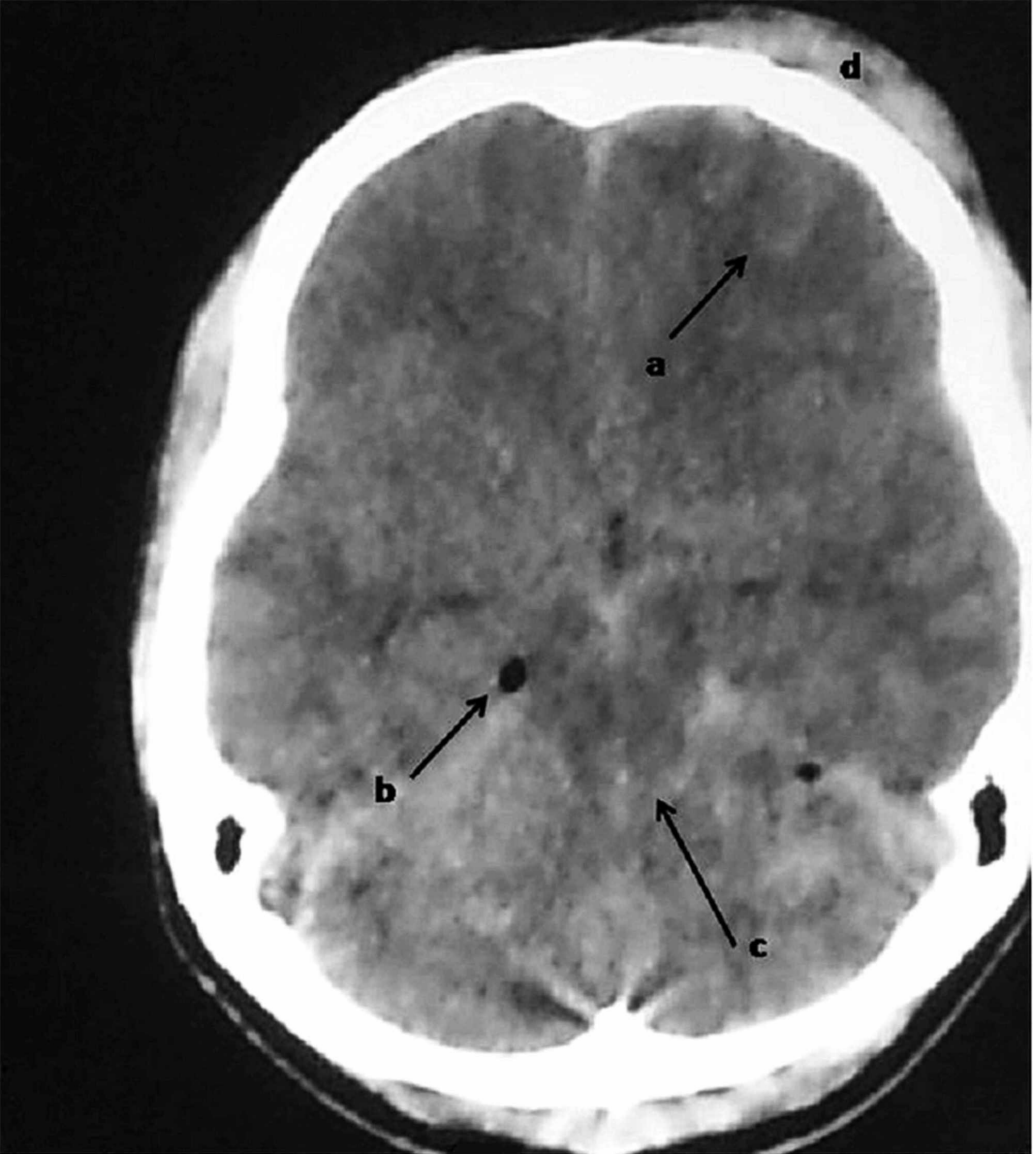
Computed tomography of the brain done at presentation (Day 1) The scan revealed: a - right frontal contusion; b - pneumocephalus; c - effacement of basal cisterns; d - right frontal subgaleal contusion
